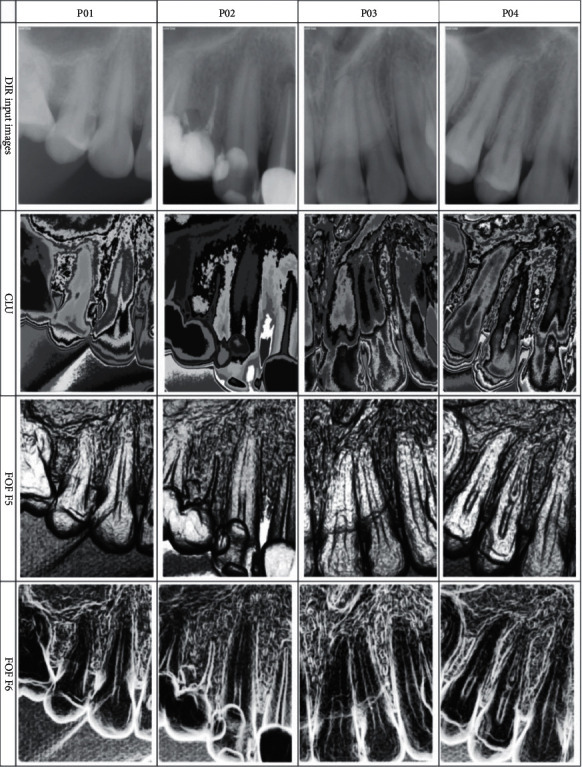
Intraoral radiographs texture feature maps [69].
